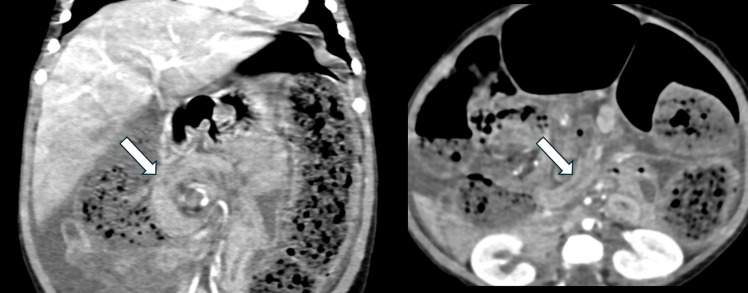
Coronal and axial CT abdomen/pelvis with IV contrast image obtained in a 10-month-old female with MMIHS who presented with lethargy and obstipation for five days. There is swirling of the mesentery (*arrows*), concerning for volvulus. At surgery, the patient was found to have malrotation with midgut volvulus. She underwent a modified Ladd procedure
